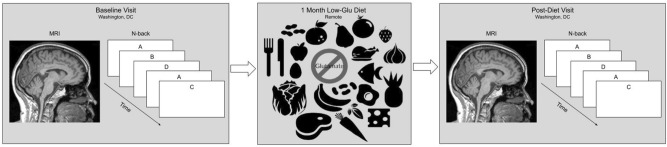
Neuroimaging study protocol. Participants came to Washington, DC for a baseline neuroimaging assessment, which included structural and functional (verbal N-back) MRI. Participants then completed detailed dietary training before being on the low glutamate diet for 1-month and returning for post-diet the structural and functional MRI.
